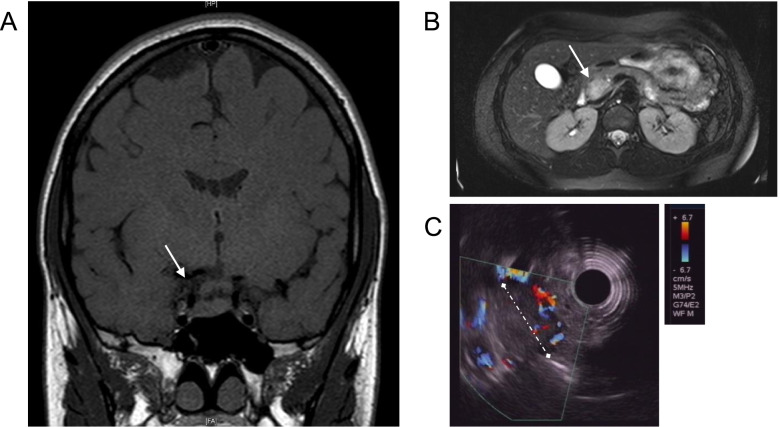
Imaging in the 28-year-old index patient. Imaging is showing an adenoma of the pituitary gland and insulinoma of the pancreas. A Magnetic resonance imaging (MRI) of the pituitary gland with tissue in lateral contact with the cavernous sinus; diameter of 5.0 × 7.5 × 4.2 mm. B T2-weighted MRI image of the abdomen documenting an inhomogeneous, hypervascularized, contrast-enhanced mass in the pancreatic head measuring 2.0 × 3.0 × 2.8 cm, consistent with an islet cell tumor. C Endoscopic ultrasound (EUS) showing color flow Doppler image of the corresponding hypervascularized lesion in the pancreatic head
